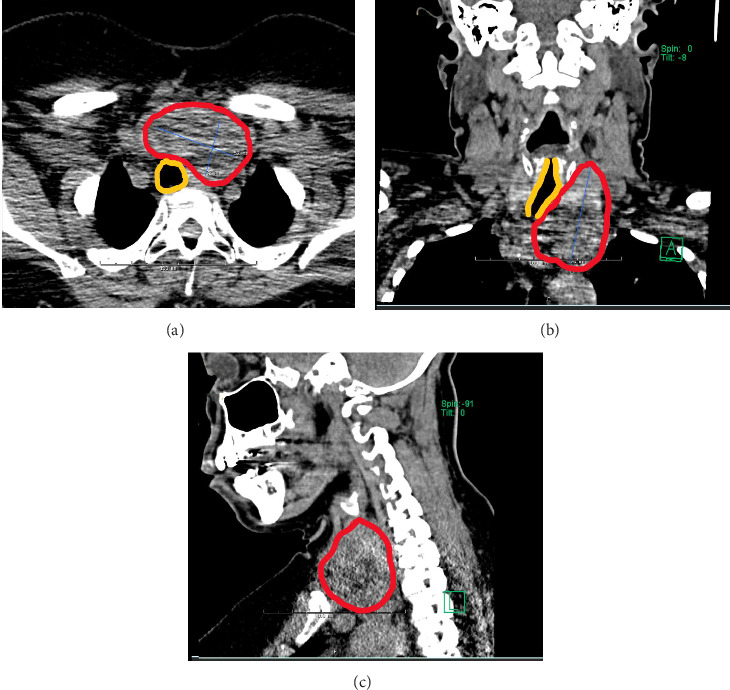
Computed tomography (CT) without IV contrast study showing thyroid nodule with substernal involvement (red outline). Trachea (yellow outline) deviated without compression due to the size and location of the nodule. (A) Axial view. (B) Coronal view. (C) Sagittal view.
